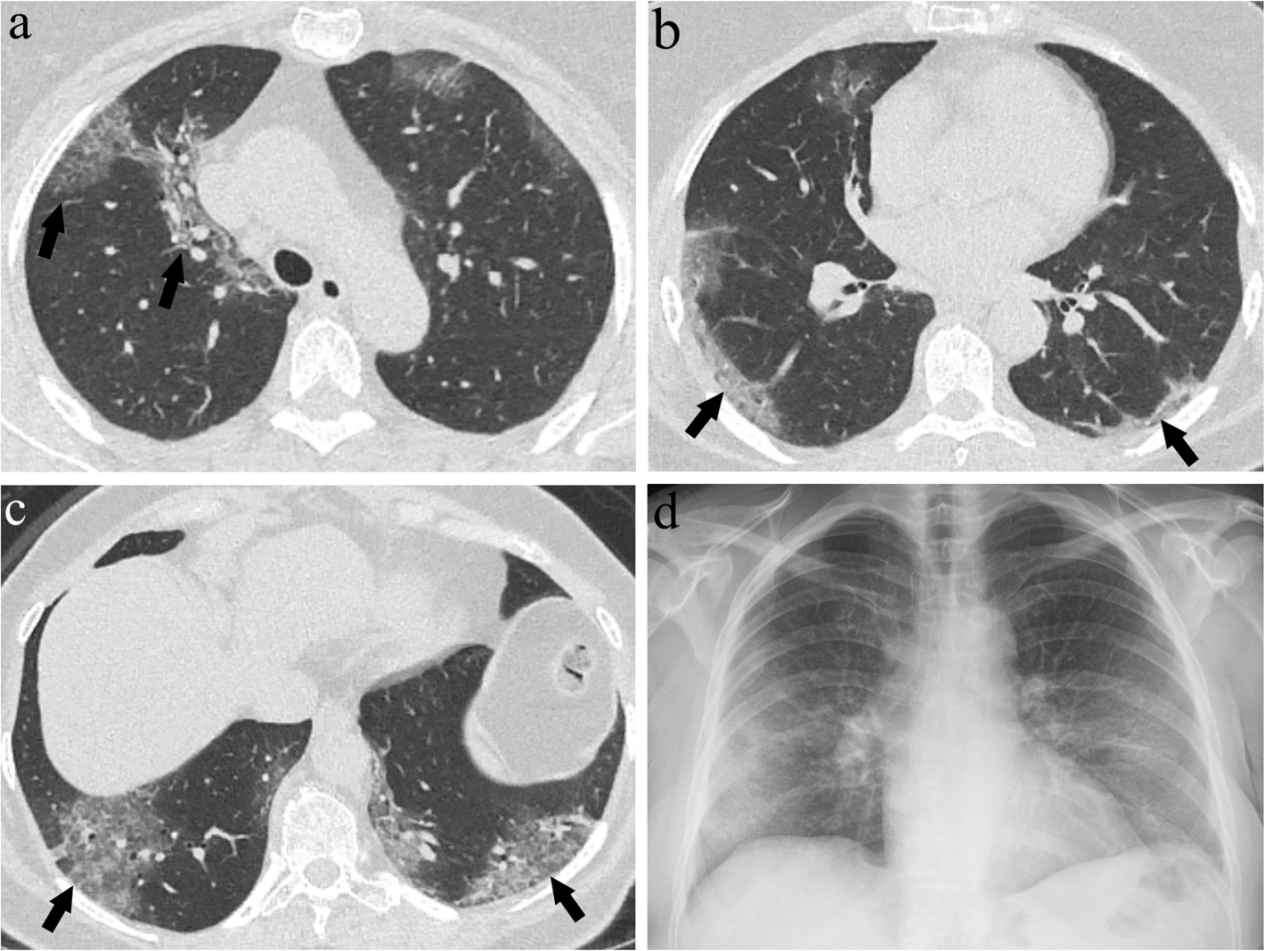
a–d A 63-year-old female presenting with mild symptoms; dry cough and fatigue. Axial CT images at the level of the upper (a), middle (b), and lower (c) lung zones show lesions predominantly distributed in peripheral areas. Additional to ground glass opacities, the crazy paving pattern and consolidations representing grade 2 and 3 lesions (arrows) were present. CXR image (d) shows peripheral hazy opacities and reticular pattern. Despite mild clinical findings, this patient had a CTS of 12 and XRS of 7 and needed monitoring in the intensive care unit during the follow-up period
